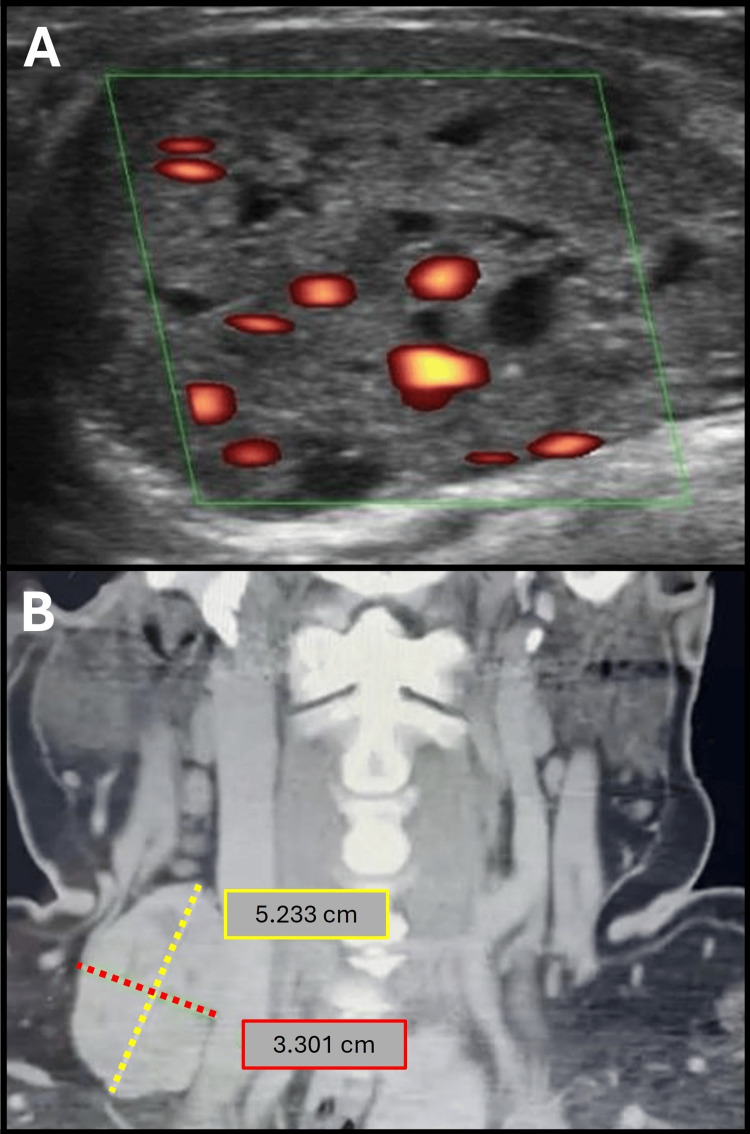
Preoperative Doppler ultrasonography and CT scan (A) Mild vascular patterns of the thyroid nodules appear on color Doppler ultrasound. (B) The coronal view shows an encapsulated mass in the right cervical lymph node, measuring 5.23 cm in length and 3.30 cm in width, with heterogeneous contrast enhancement.
